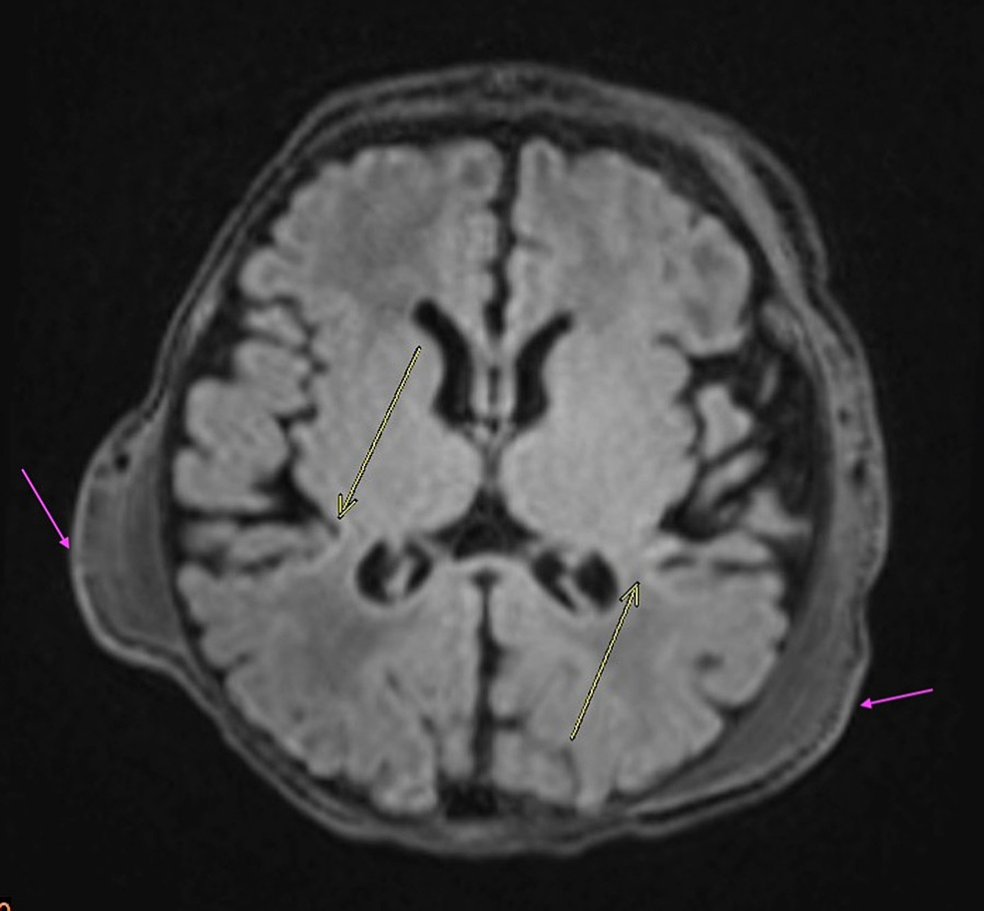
Axial T2 FLAIR demonstrating subtle increased intensity involving posterior insular cortices bilaterally (yellow arrows) along with bilateral parietal cephalohematomas (purple arrows). FLAIR: Fluid-attenuated inversion recovery
